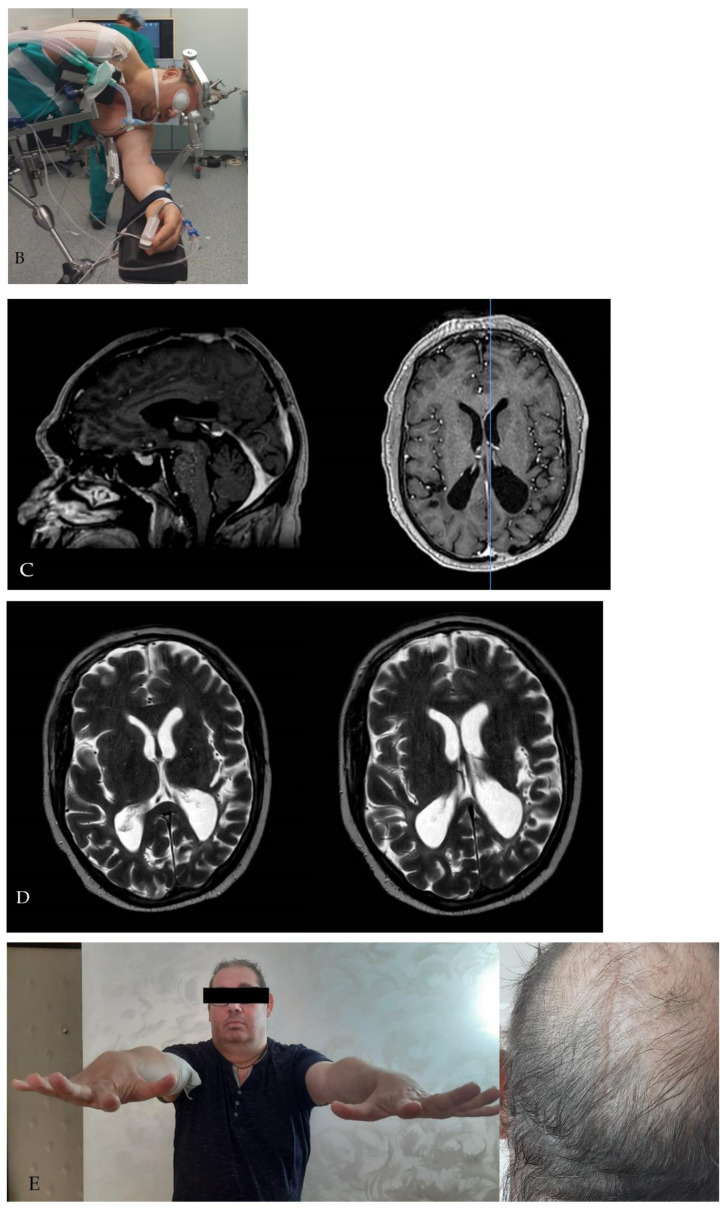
(A) Pre-operative MRI documenting the presence of a lesion partially involving the left atrial cavity of the lateral ventricle. (B) Three-quarter prone position for the IITA. (C) Immediate post-operative MRI (T1 sequence with cm) control documenting the complete resection of the lesion and the rehabilitation of the atrial cavity. (D) Immediate post-operative MRI (T2 sequence). Note the absence of post-surgical brain edema. (E) One month post-operative neuological evaluation and surgical linear incision aspect.
